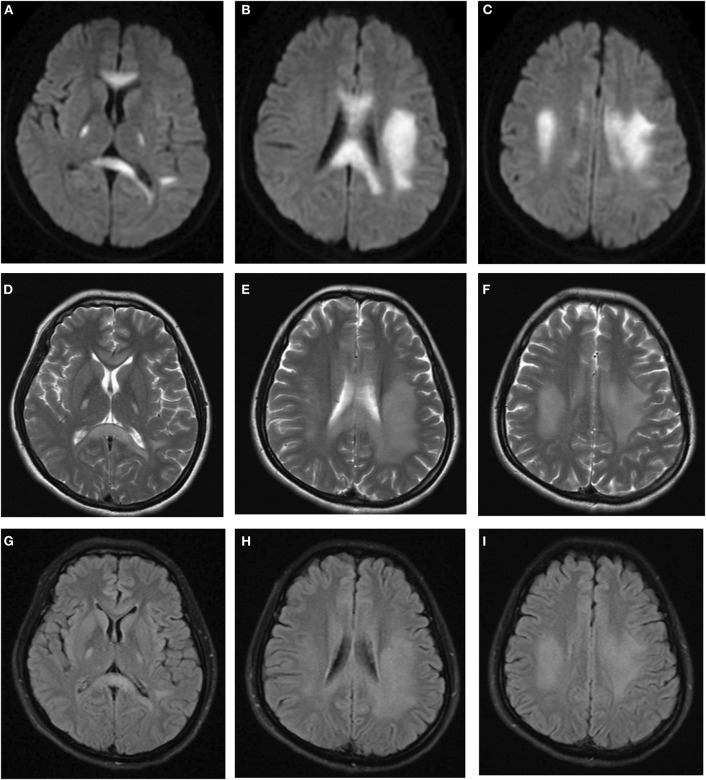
Brain MRI indicated diffuse abnormal signals in corpus callosum, posterior limbs of bilateral internal capsule, and centrum semiovale. (A–C) Diffusion weighted imaging. (D–F) T2-weighted imaging. (G–I) FLAIR imaging.
